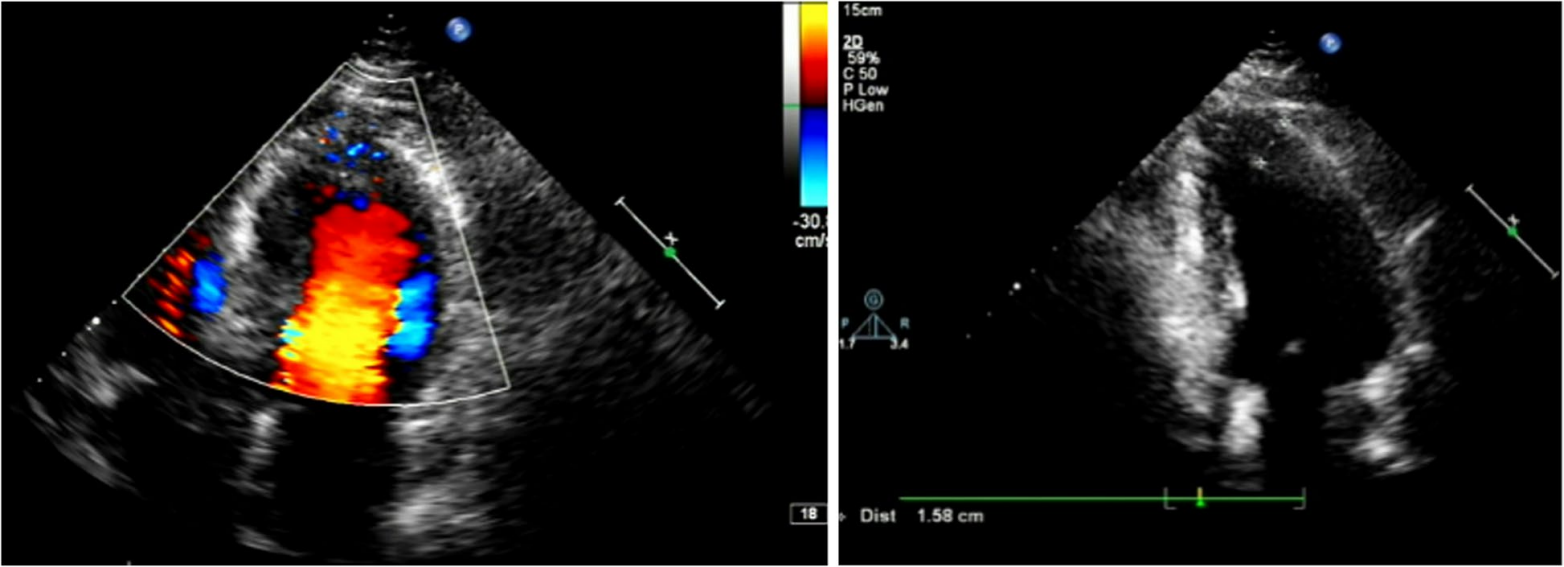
Abnormal manifestations of the patient's echocardiography. (B-1) Color-flow Doppler Imaging showed the blood flow signal was visible in the apical segment wall(yellow triangle), which was more obvious in the subepicardial, and the subendocardial blood flow was connected to the left ventricle. (B-2). Apical myocardium markedly thickened.
